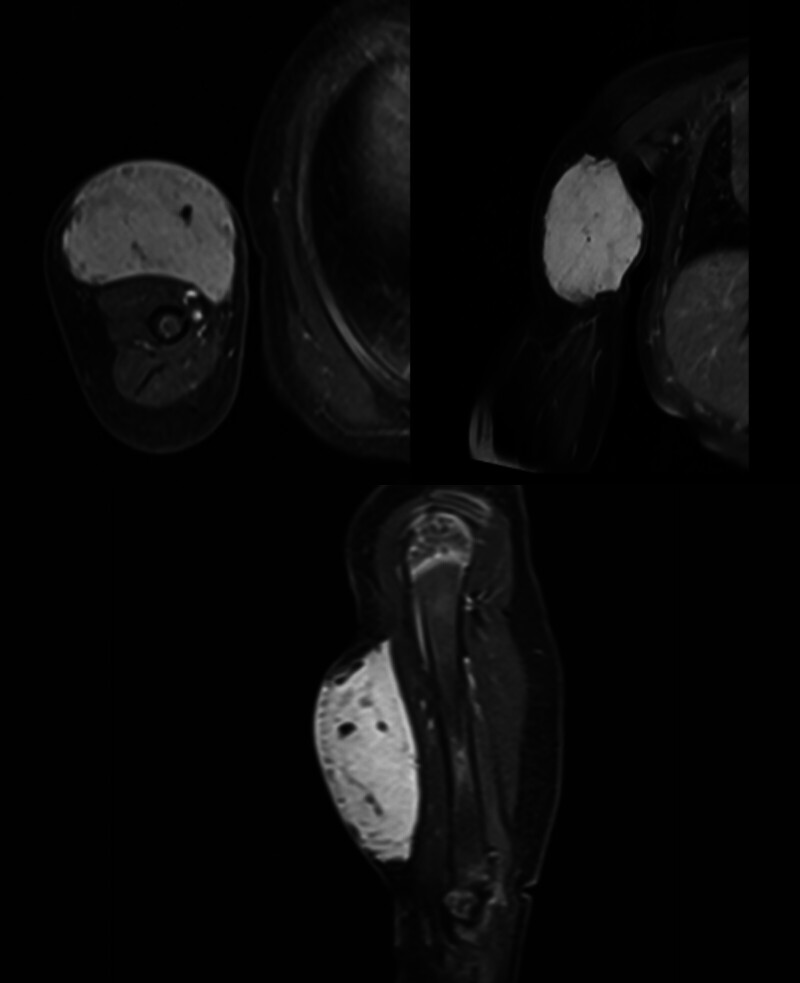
Axial, sagittal, and coronal post-contrast fat-suppressed T1-weighted MR images of the right arm demonstrating a well-defined subcutaneous fusiform lesion with vivid heterogeneous enhancement. No frank intramuscular extension or cortical invasion is identified.
